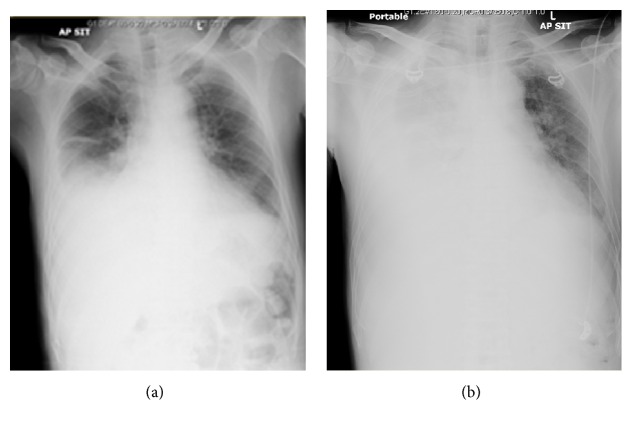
Chest radiographs showing a right pleural effusion on admission (a) with worsening of the effusion one day after (b).
